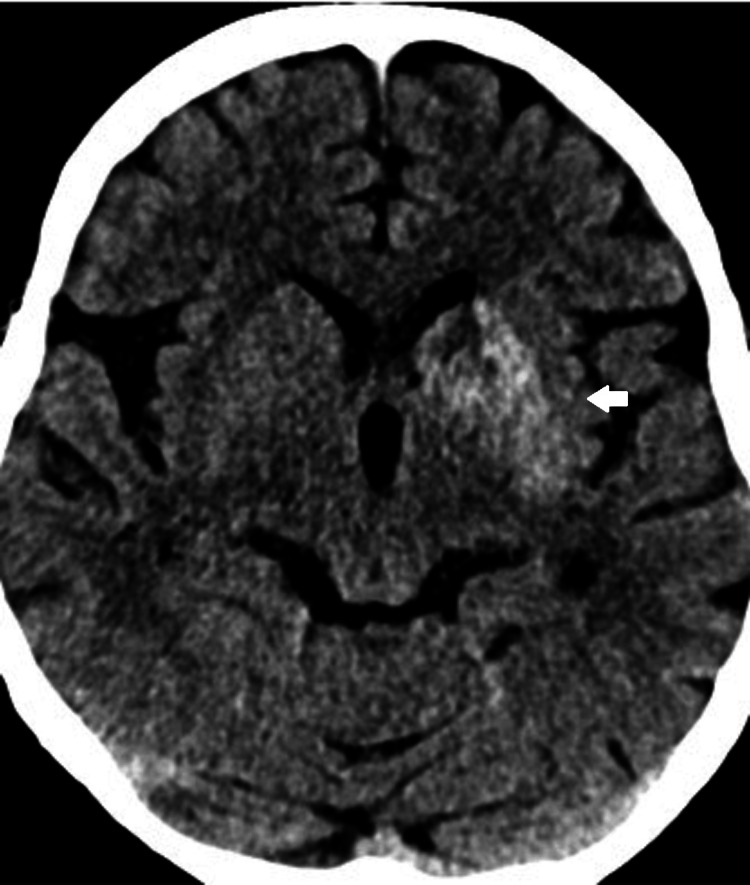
Non-contrasted CT brain showed hyperdensity at the left basal ganglia
